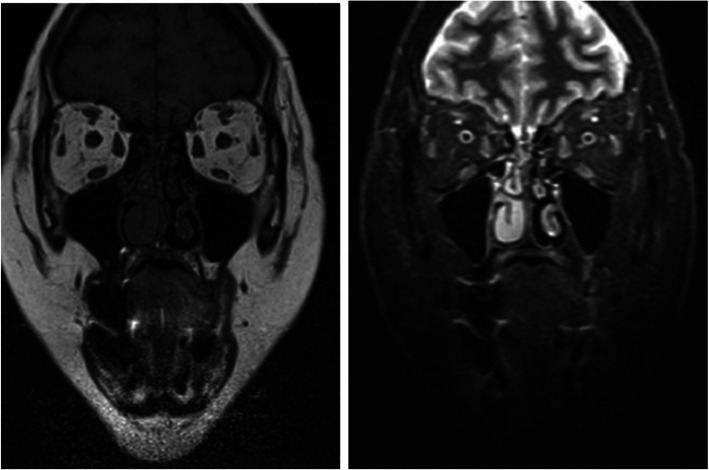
Follow-up orbital MRI performed upon completion of oral therapy; showing resolution of the edematous anomaly of the extraocular muscles and regression of proptosis to the right
